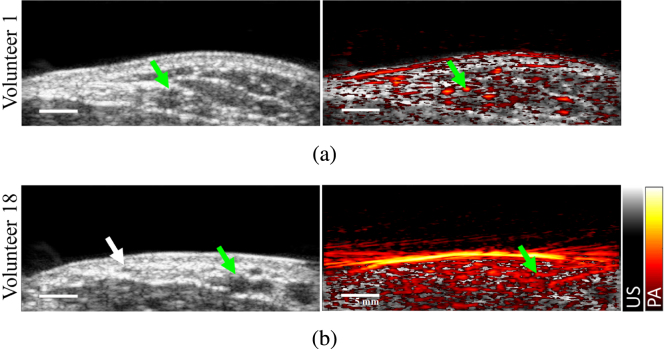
Ultrasound B-mode images (left) and B-mode overlaid by conventional amplitude-based PA image at 810 nm (right) of (a) volunteer 1 (lightest skin tone) and (b) volunteer 18 (darkest skin tone). The green arrows indicate the RA location. The white arrow indicates the location of a smaller blood vessel visible in the ultrasound B-mode image.
